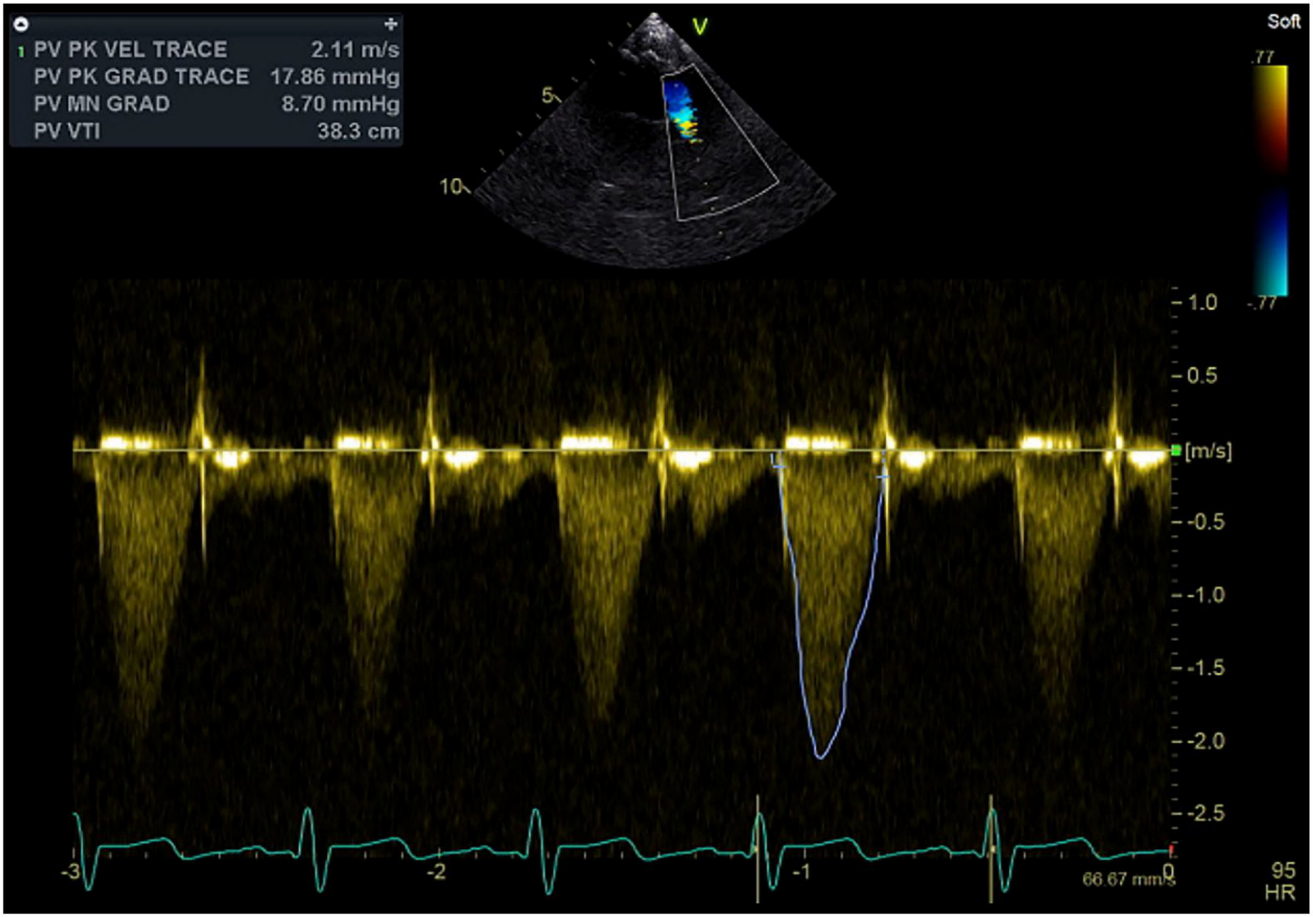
Continuous wave Doppler signal with the sample volume at the level of the pulmonary outflow tract, neo-pulmonary valve, and pulmonary trunk demonstrating well-functioning valve and absence of obstruction.
